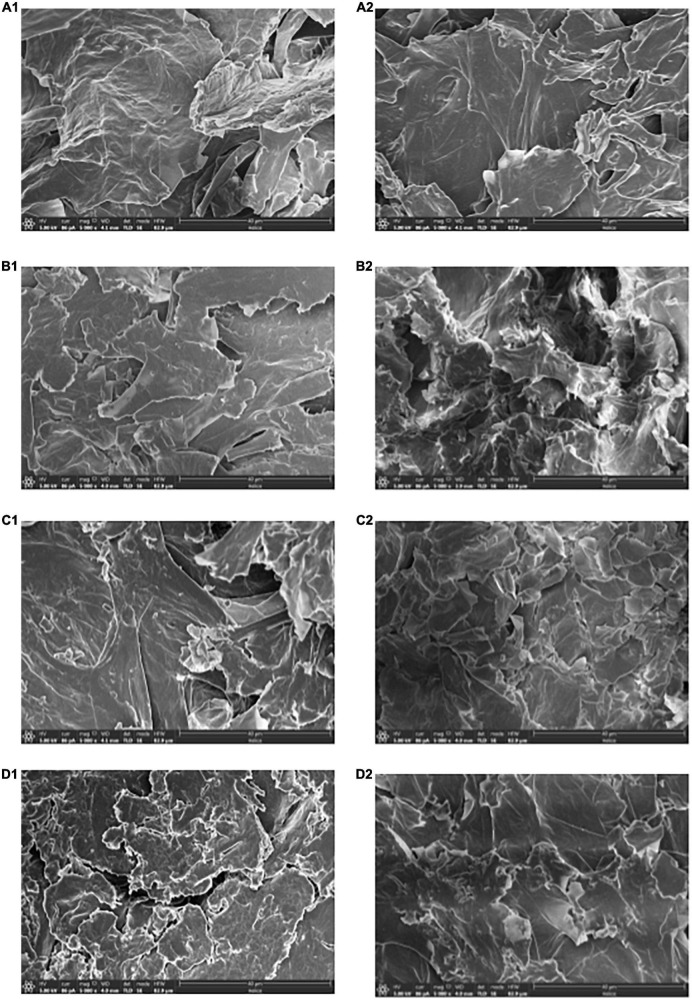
SEM images of *Pleurotus eryngii* chips before frying (A1–D1) and after frying (A2–D2): (A) Blanching; (B) Blanching + osmosis; (C) Blanching + ultrasound; (D) Blanching + ultrasound + osmosis.
